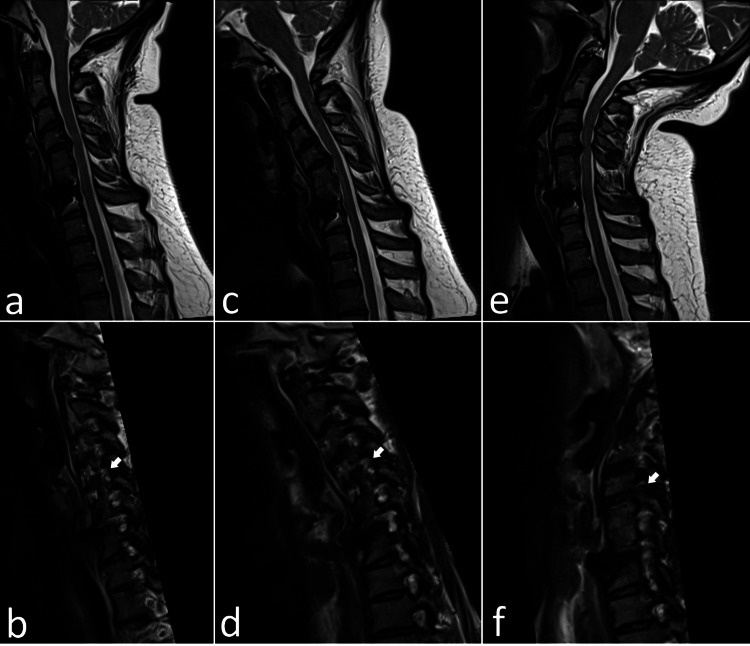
T2-weighted MRI images of the cervical spine in sagittal and right parasagittal views during (a, b) neutral position, (c, d) flexion, and (e, f) extension. In the extended position, there is increased disc protrusion along with posterior ligamentous buckling, resulting in severe spinal canal stenosis at the C4-C5 level. Neck extension also leads to marked stenosis of the right C4-C5 neuroforamen (arrows).
